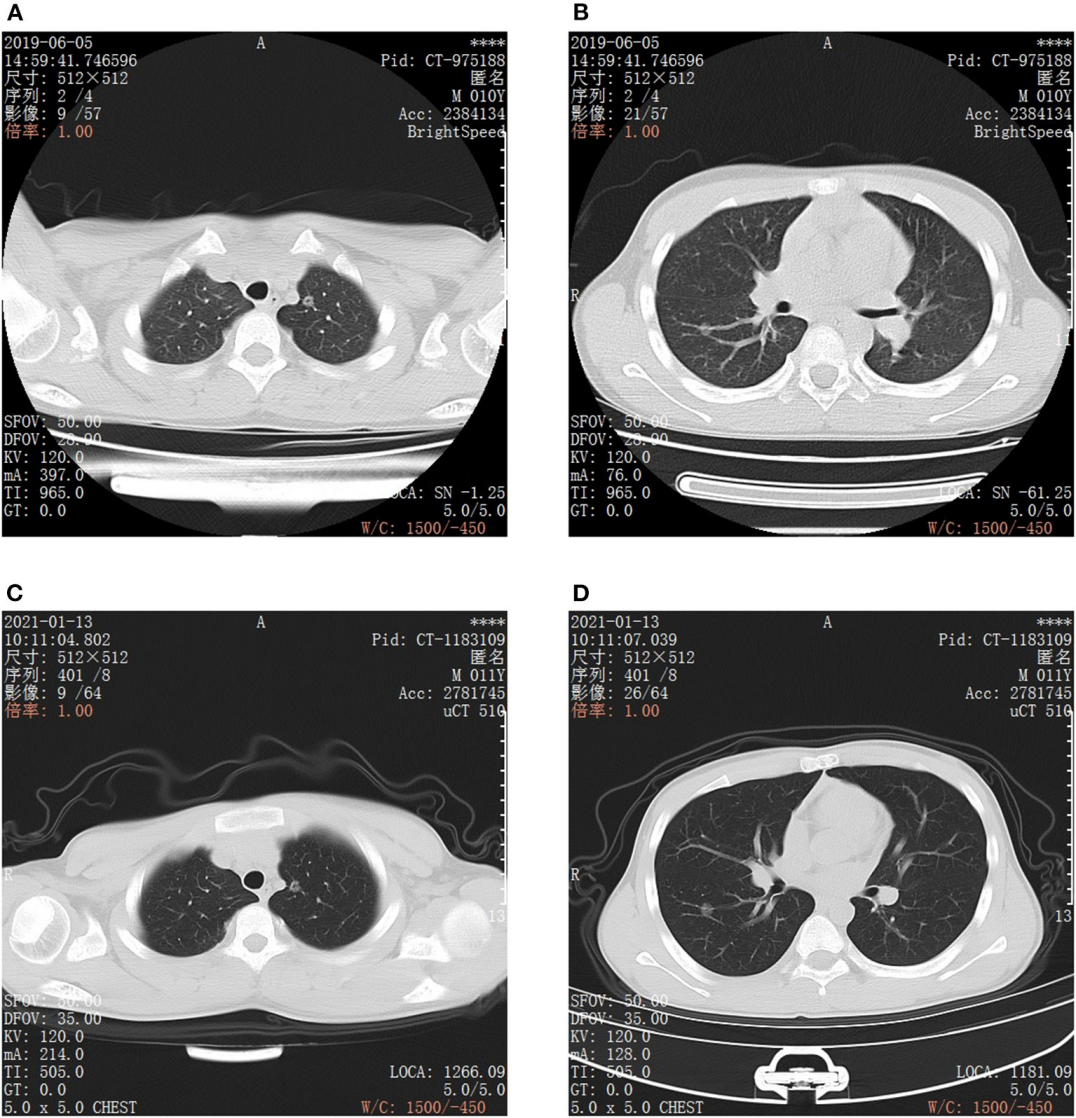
CT images of bilateral chest. (A,B) are CT scan images taken on June 5, 2019, Scanning model: GE BrightSpeed, scanning parameters: slice thickness: 5 mm, gap: 5 mm. (A) Round ground glass opacity in the posterior segment of the left superior lobe apex, the boundary is clear, in the center, there is a dot-like translucent shadow. (B) Round ground glass opacity in the anterior basal segment of the right lower lobe, the edge is smooth and clear, it can be seen that vascular shadow naturally passes through. (C,D) are CT scan images taken on January 13, 2021, scanning model: United image uCT, scanning parameters: slice thickness: 5 mm, gap: 5 mm. The 2 ground glass opacities of the posterior segment of the left upper lobe apex (C) and the anterior basal segment of the right lower lobe (D) are roughly similar to that of the film taken on June 05 2019. (C) CT image of ground glass opacity in the posterior segment of the left superior lobe apex. (D) CT image of ground glass opacity in the anterior basal segment of the right lower lobe.
